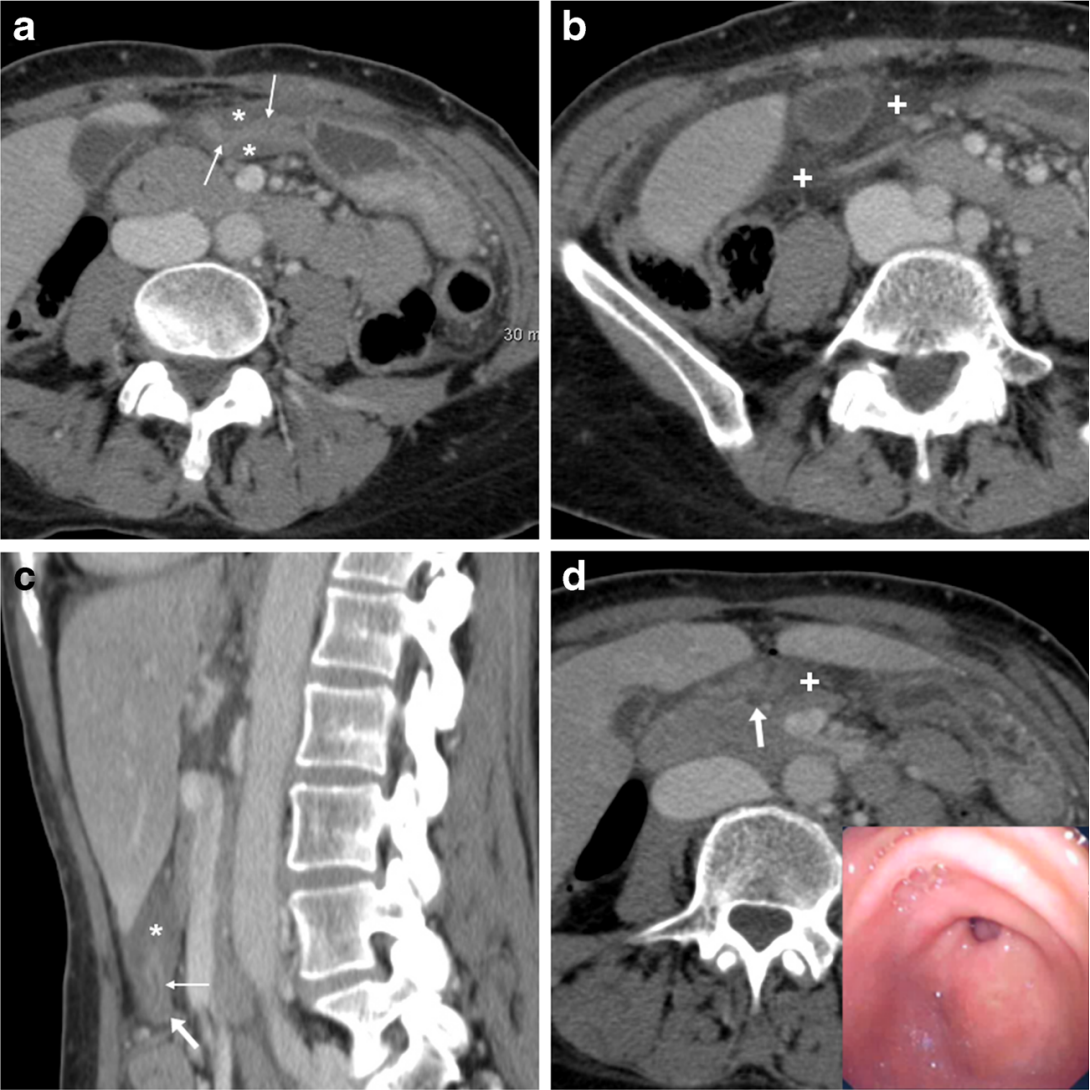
A 55-year-old female with unremarkable medical history experienced severe abdominal pain with left-sided tenderness and minimally elevated serum lipase. Multiplanar CT images showed collapsed gastric antrum with moderately thickened, stratified wall (*) and enhancing mucosa (thin arrows). Additionally, inflammatory hyperattenuation of surrounding and infrahepatic fat planes (+) reinforced suspicion of acute PUD. The subtle, small-sized mucosal defect with centimetric outpouching (arrows in c, d) corresponded to endoscopic finding (image in inset d) of posterior prepyloric ulcer with oedematous margins and fibrinous crater. The patient received medical therapy with continuous PPI infusion
